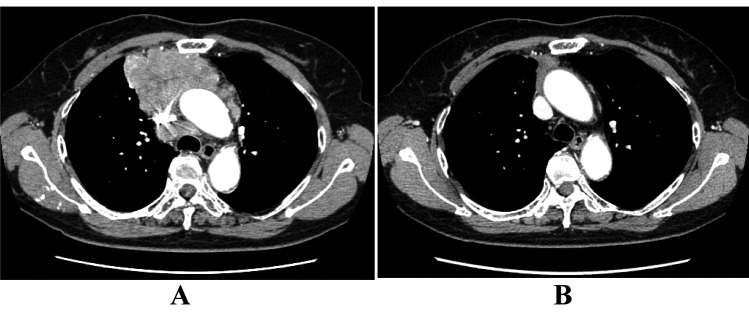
The response to initial ADOC chemotherapy in case 1. Chest computed tomography before initial ADOC chemotherapy (A) and after four cycles of initial ADOC chemotherapy (B).
